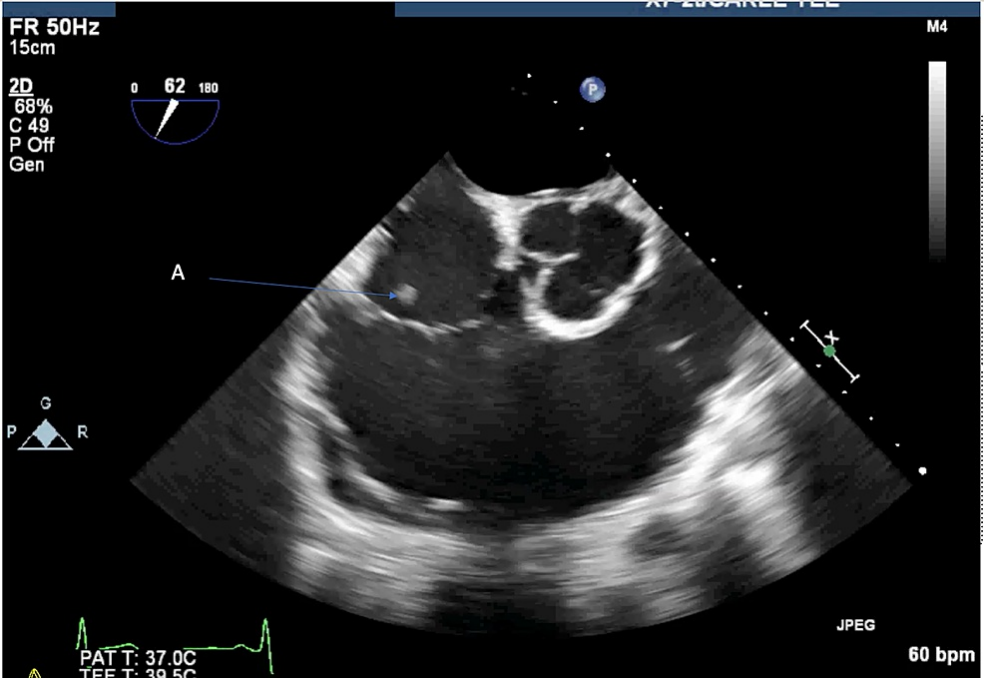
Transesophageal echocardiogram showing tricuspid valve endocarditis Transesophageal echocardiogram showing tricuspid valve endocarditis with moving into the right atrium with valve closure [A].
